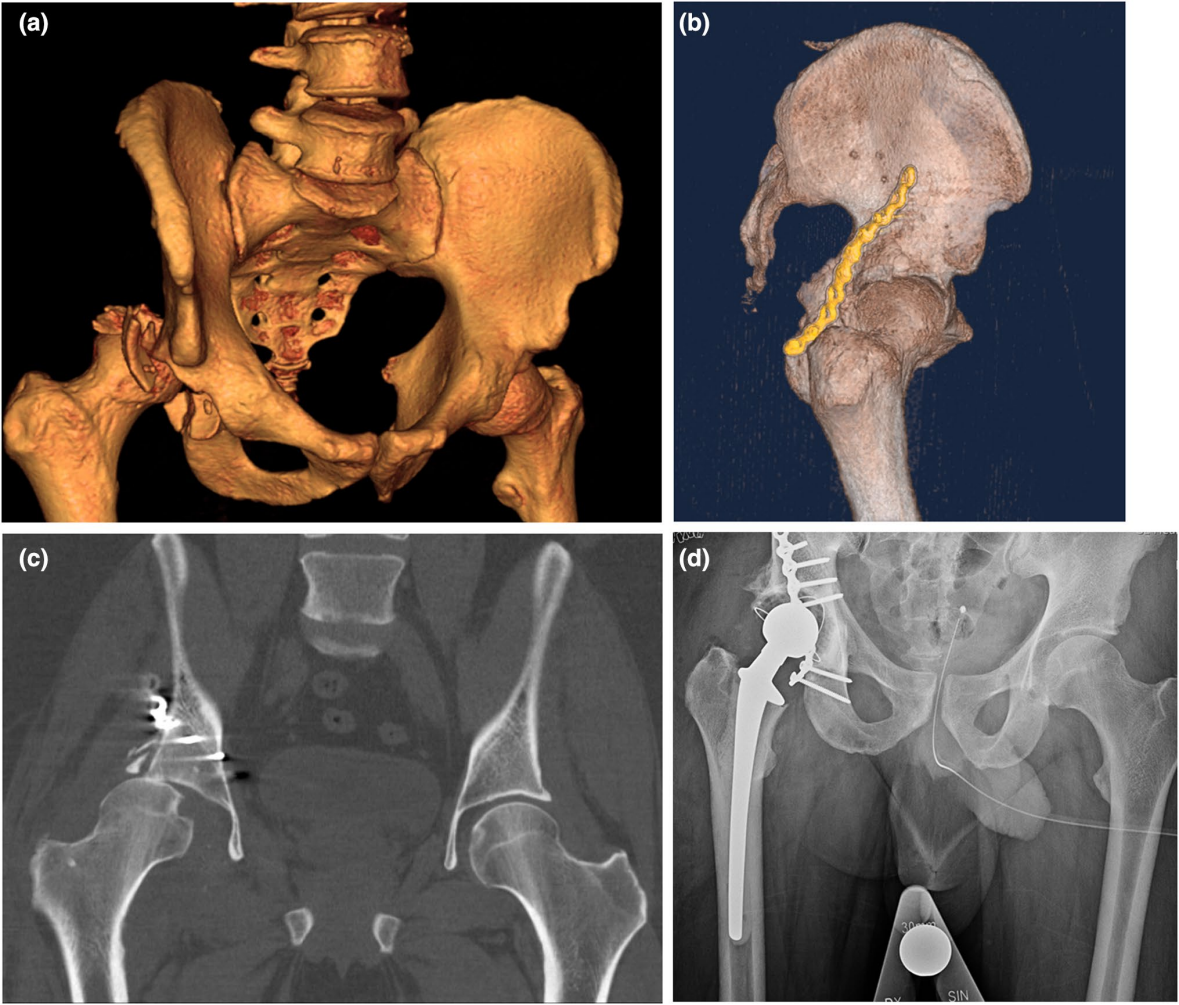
a Computed tomography (CT) scan of a 45-year-old male who sustained a posterior wall acetabular fracture with dislocated hip after a high energy car accident. b Postoperative CT scan after treatment with plate fixation. c CT scan after 6 weeks showing osteosynthesis failure with subluxation of the femoral head. d Postoperative radiographs after reoperation with a cemented THA
